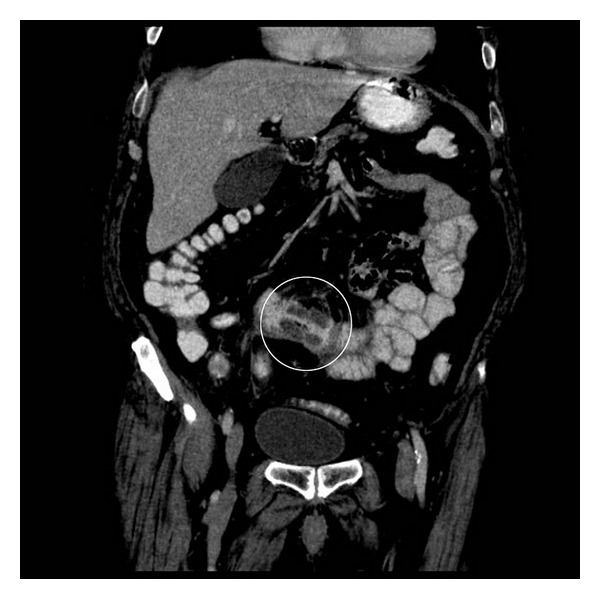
Apple core lesion in small bowel, see circle (computed tomography).
